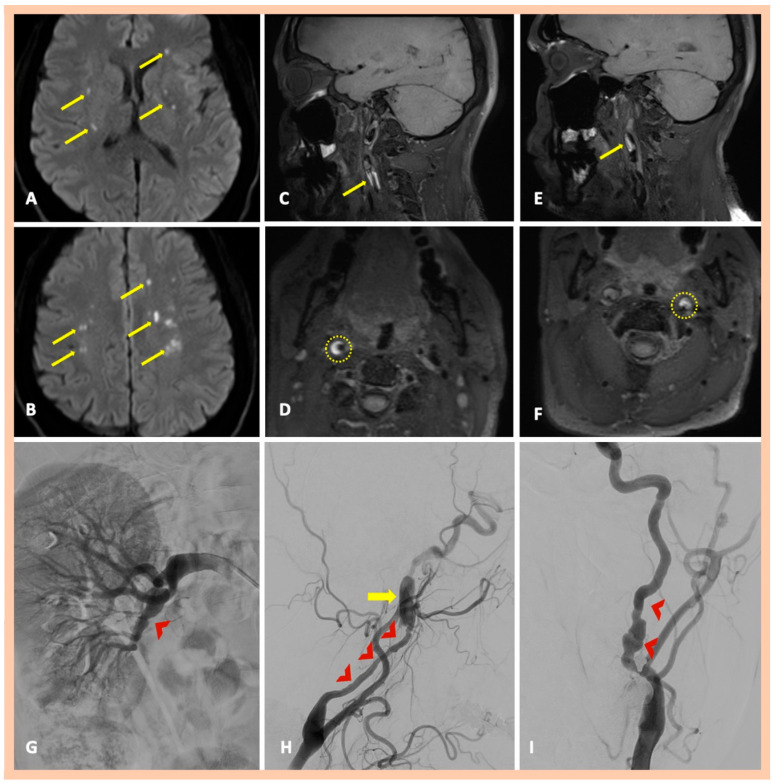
Neuroimaging findings—Patient #7. DWI sequence revealing multiple acute ischemic lesions with diffusion restriction in multiple arterial territories bilaterally (Panels (A,B)). Three-dimensional fat-saturated black-blood T1-weighted high-resolution (3 Tesla) vessel wall imaging with multiplanar reconstruction, showing crescent-shaped, hyperintense lesions in the vessel wall of the right ICA (Panels (C): Sagittal—(D): Axial) and left ICA as well (Panels (E): Sagittal—(F): Axial), indicating intramural hematoma along both arteries. DSA revealing the characteristic focal stenosis of the right renal artery (Panel (G)) and the characteristic “string-of-beads” appearance along the extracranial segments of the right (Panel (H)) and left ICA (Panel (I)) with the presence of a dissecting aneurysm along the distal cervical and proximal petrous artery segments of the right ICA (Panel (H); yellow arrow).
